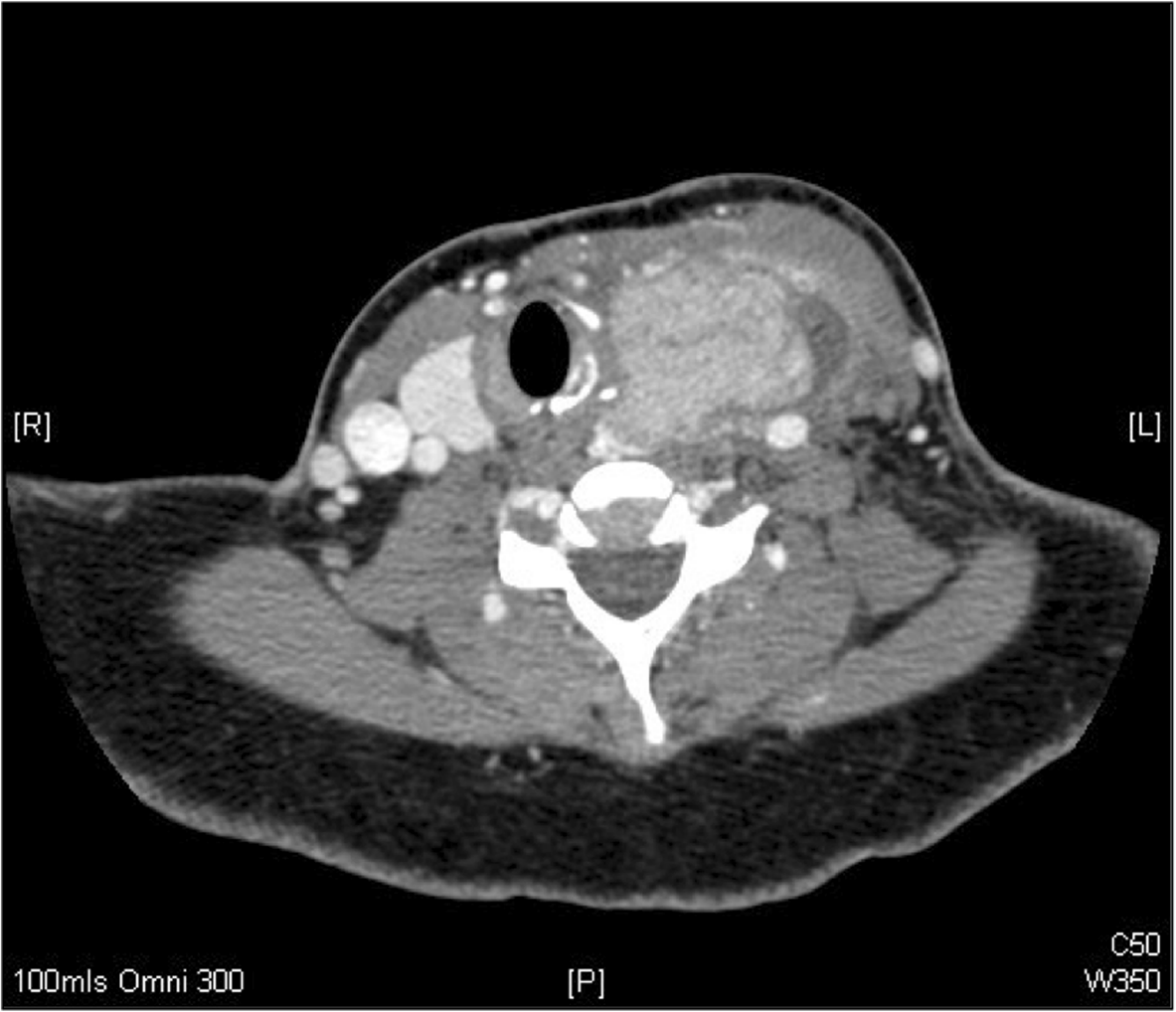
CT with contrast axial slice through neck. A more superior CT neck slice demonstrating a resolving abscess and concurrent left thyroid soft tissue mass, now confirmed as a papillary thyroid carcinoma.
